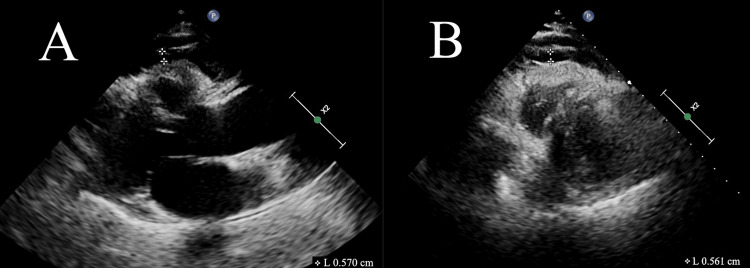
Echocardiographic measurement of EAT thickness in the parasternal long-axis view (A) and parasternal short-axis view (B) of the left ventricle at end-systole from an RA patient. Abbreviations: EAT, epicardial adipose tissue; RA, rheumatoid arthritis
